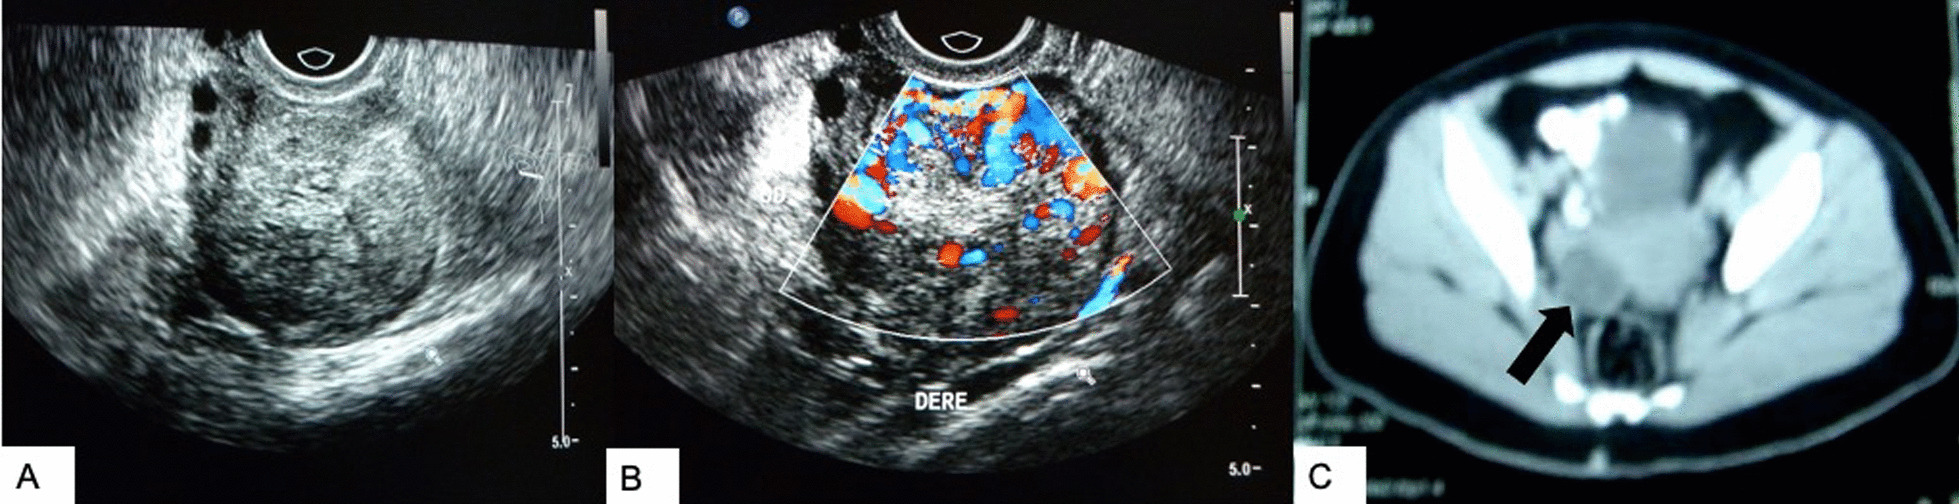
Ultrasound and CT scans of the patient. A, B Endovaginal ultrasound. We observed an enlarged right ovary due to a heterogeneous solid tumor with different echogenic areas. This area has an increased vascularity with the application of Doppler. C Axial slices in CT. The arrow is pointing to a hypodense solid lesion in the enlarged right ovary
